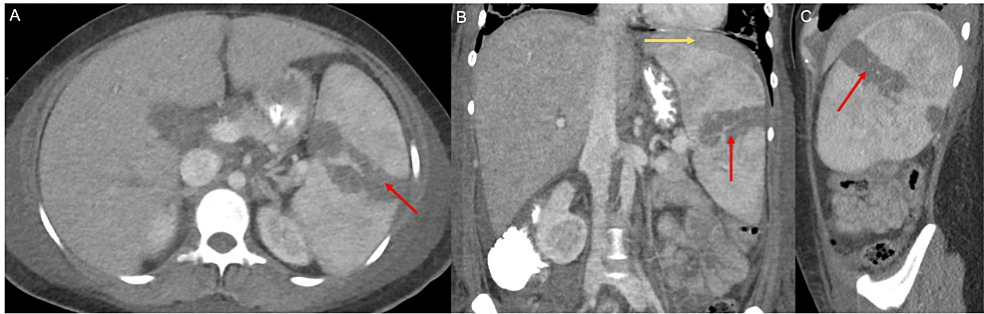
Axial (A), coronal (B), and sagittal (C) CT images of the abdomen with intravenous contrast demonstrate a 6.9 cm linear hypodensity in the splenic parenchyma (red arrow in A, B, and C) with a small amount of perisplenic fluid (yellow arrow in B), consistent with a grade 3 laceration.
